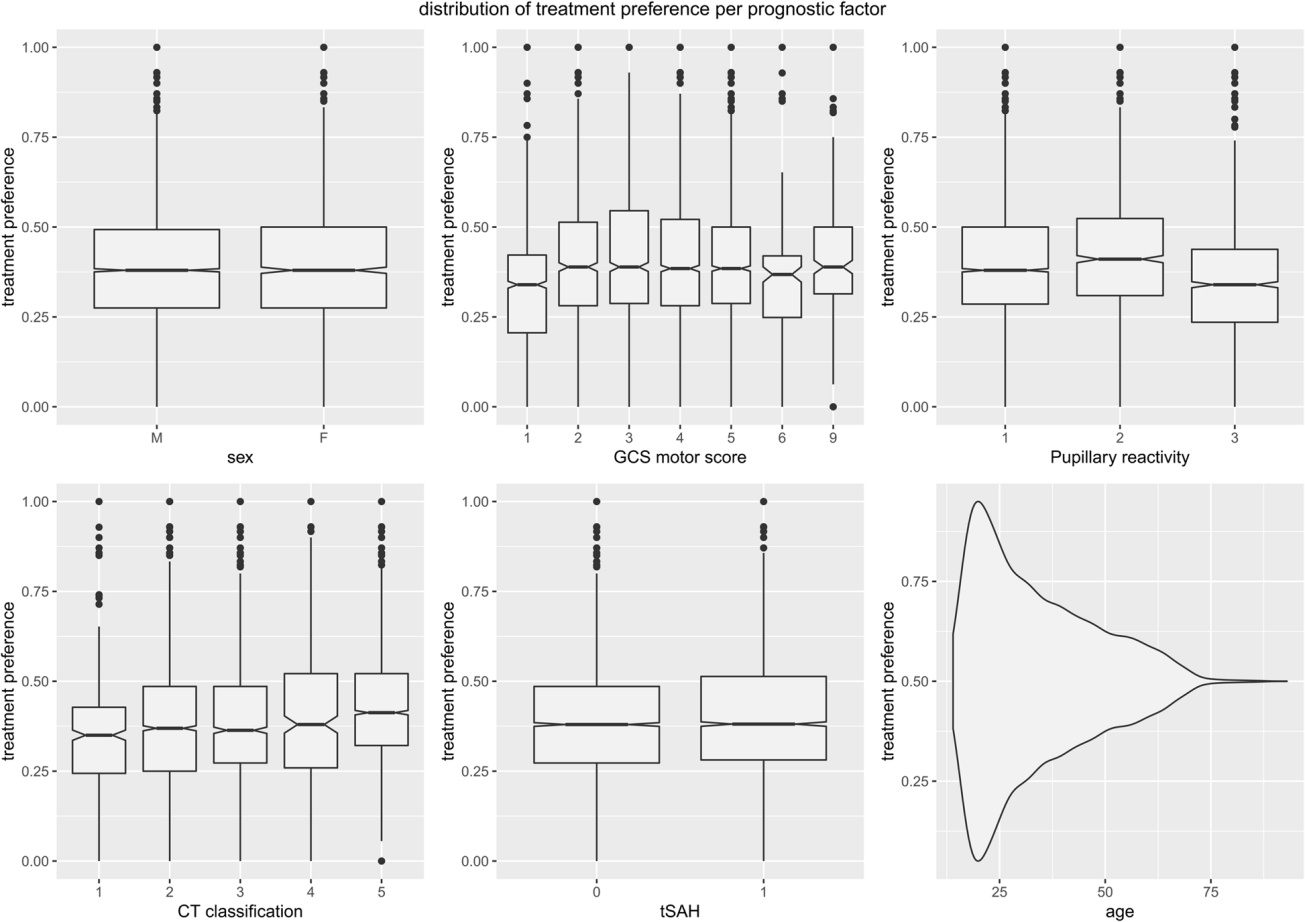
Distribution of the IV (treatment preferences) plotted per prognostic factor in the motivating example showing the distribution of the treatment preference of the hospitals attributed to each patient, per level of the prognostic factor: sex, Glasgow Coma Scale (GCS) motor score, pupillary reactivity, the Traumatic Coma Data Bank computed tomography (TCDB CT) scan classification, the presence of subarachnoid hemorrhages (SAH) and age. CT classification is based on the Marshall classification
